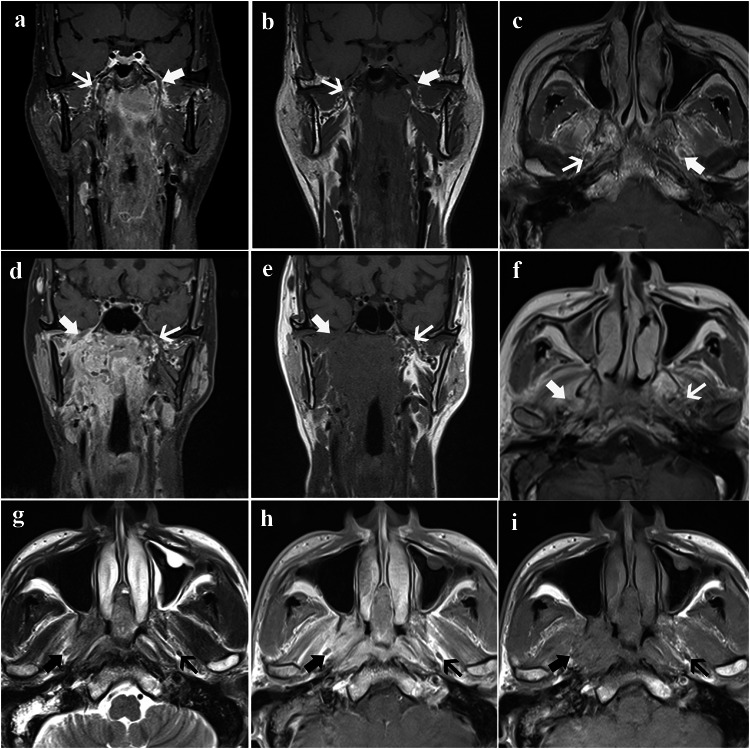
Magnetic resonance images in three cases with mandibular nerve involvement. Case 1: a Enhanced coronal, b coronal, and c axial T1-weighted images in a 47-year-old man show the enlargement of the mandibular nerve (thick arrow) and normal mandibular nerve (thin arrow). Case 2: d Enhanced coronal, e coronal, and f axial T1-weighted images in a 77-year-old female show the enlargement and abnormal enhancement of the mandibular nerve (thick arrow) and normal mandibular nerve (thin arrow). Case 3: g Axial T2-weighted image, h axial, and i enhanced axial T1-weighted images in a 58-year-old man show the enlargement of the mandibular nerve (thick arrow) and normal mandibular nerve (thin arrow). In all three patients, abnormal enhancement of the soft tissue along mandibular nerve was observed
